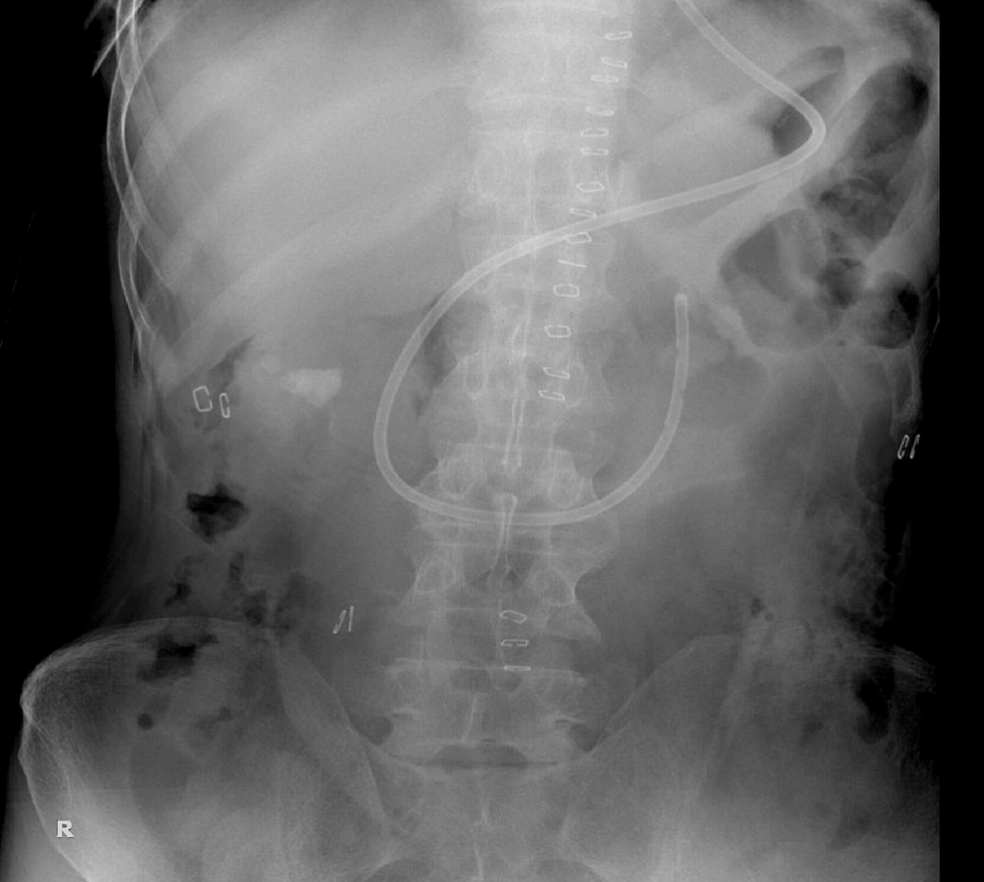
X-ray abdomen confirming the correct position of NJT at the end of the procedure. NJT, nasojejunal tube
